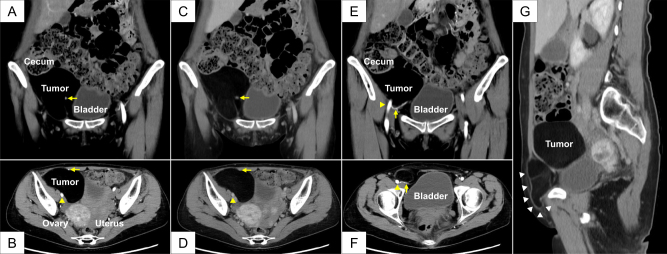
Contrast-enhanced CT findings of the pelvic mass. Coronal (A) and axial (B) images in the early phase, and coronal (C) and axial (D) images in the delayed phase show a well-defined retroperitoneal mass located anterior to the external iliac vessels, compressing the adjacent structures without internal enhancement. Early-phase coronal (E) and axial (F) images show the inferior epigastric vessels located caudal to the tumor. Yellow arrowheads and arrows, respectively, indicate the right external iliac artery and the inferior epigastric artery. White arrowheads in (G) indicate the tumor prolapsing into the inguinal canal.
